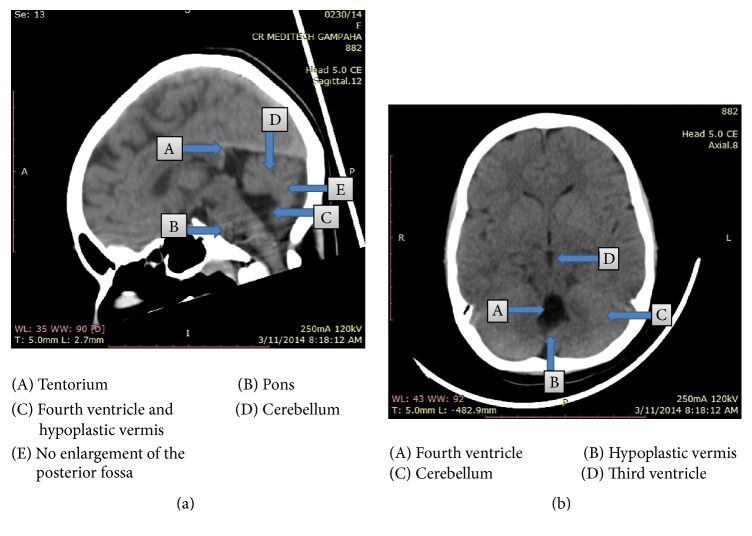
CT images of the patient, showing Dandy-Walker malformation.
